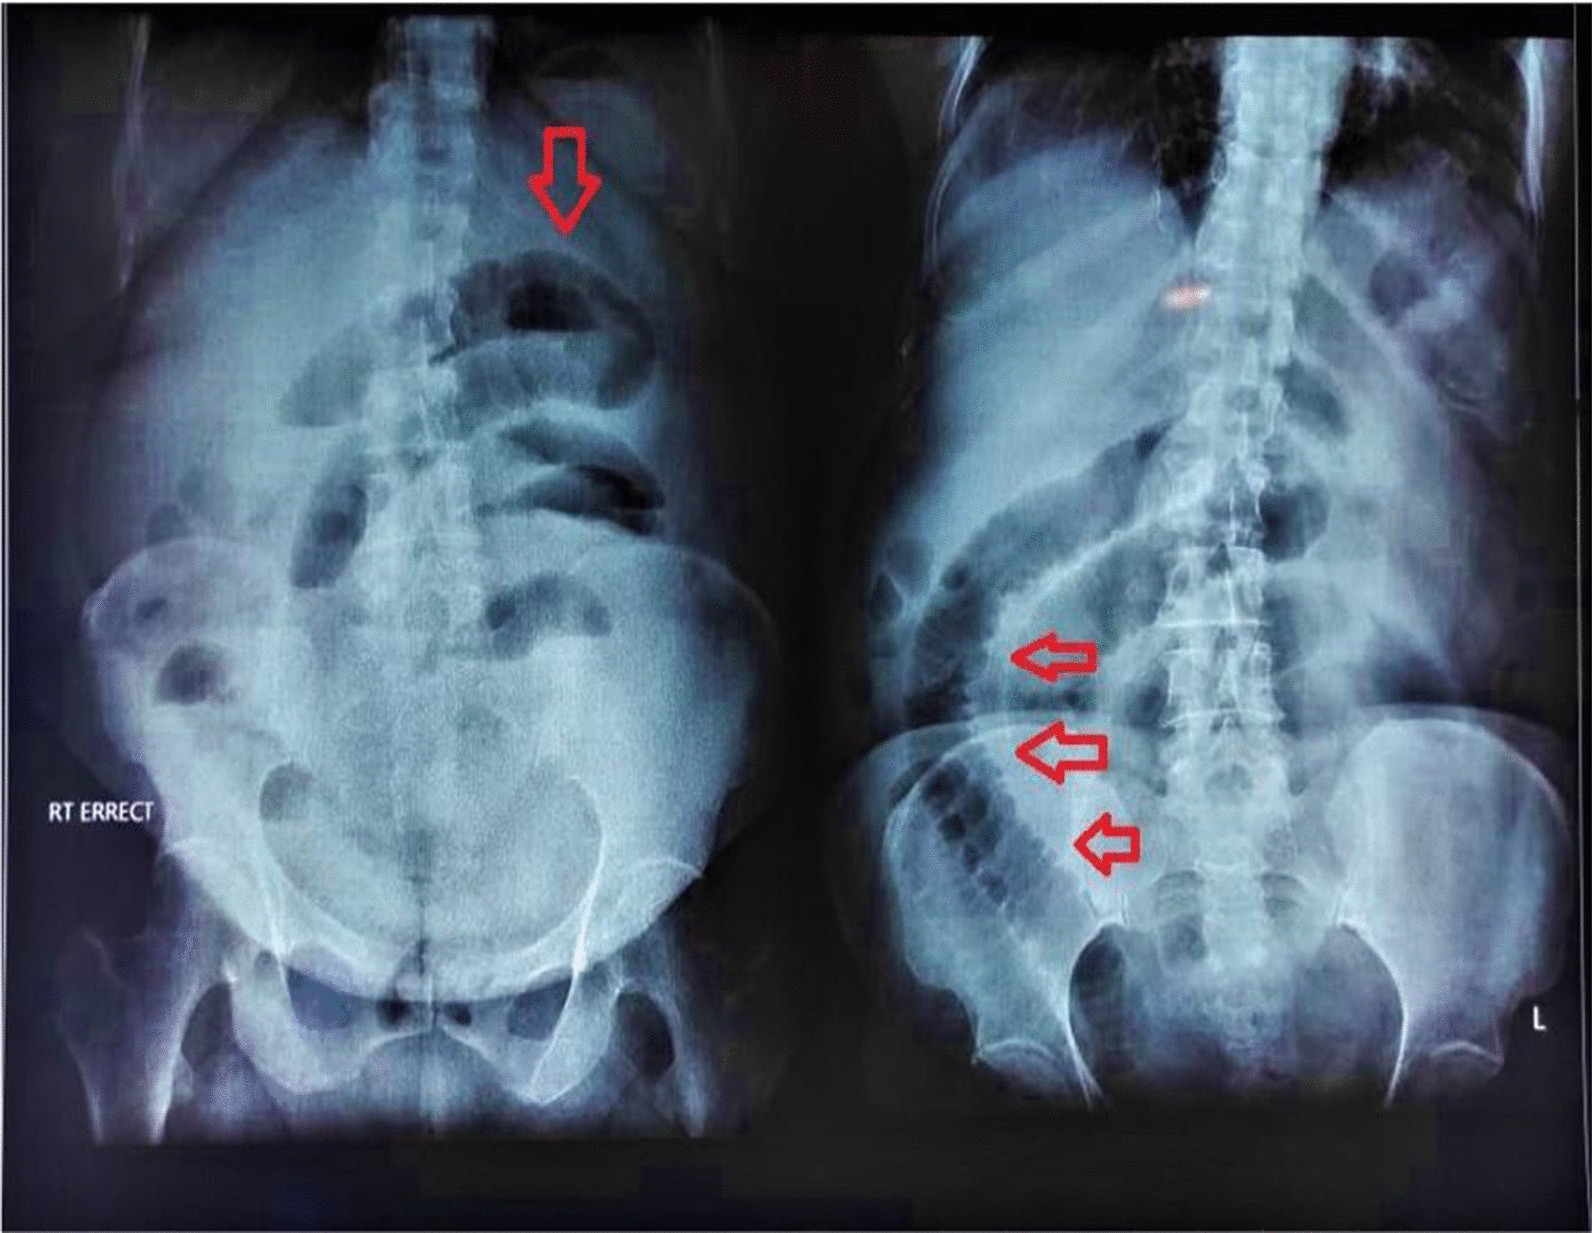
Erect x-ray abdomen showing valvulae conniventes and featureless ileum and dilated small bowel loops. Red arrows shows valvulae conniventes and featureless ileum and dilated small bowel loops
